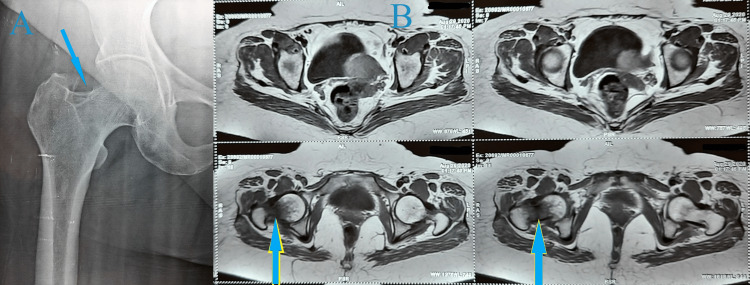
A) Right hip X-ray showing a nondisplaced stress femur neck fracture on the tension side of the cortex. B) MRI showing bone edema and stress fracture of the right femur neck
